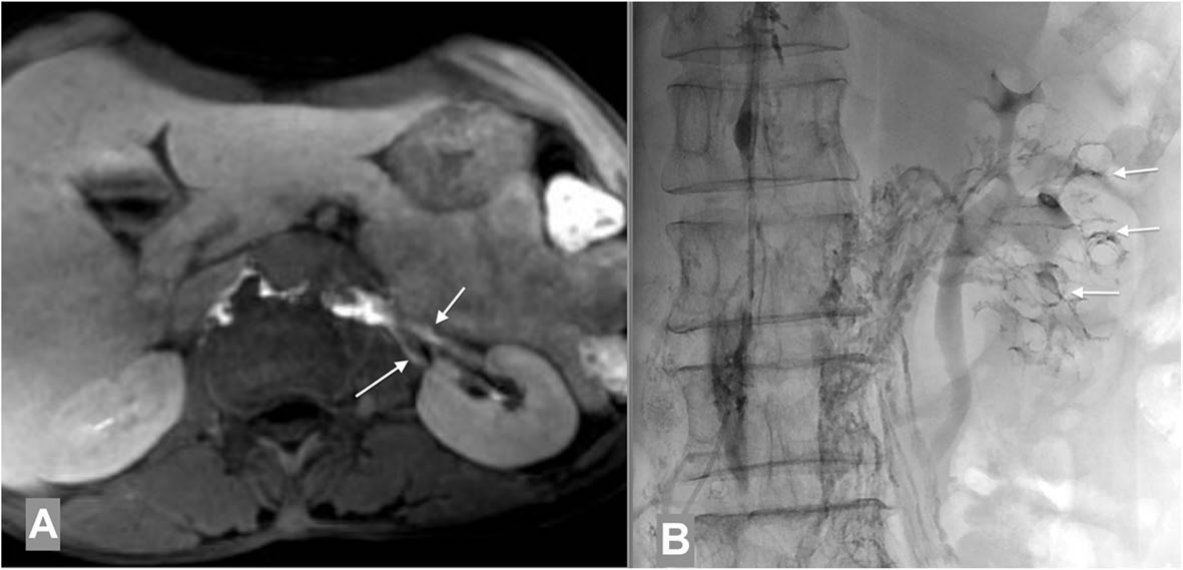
Abnormal lymphatic vessels on the left kidney. A Renal – lymphatic fistular on the left kidney seen on MRI (arrows). B Multiple tiny lymphatic vessels toward the left kidney and the fistula into the renal collecting system (arrows) seen fluoroscopic image
